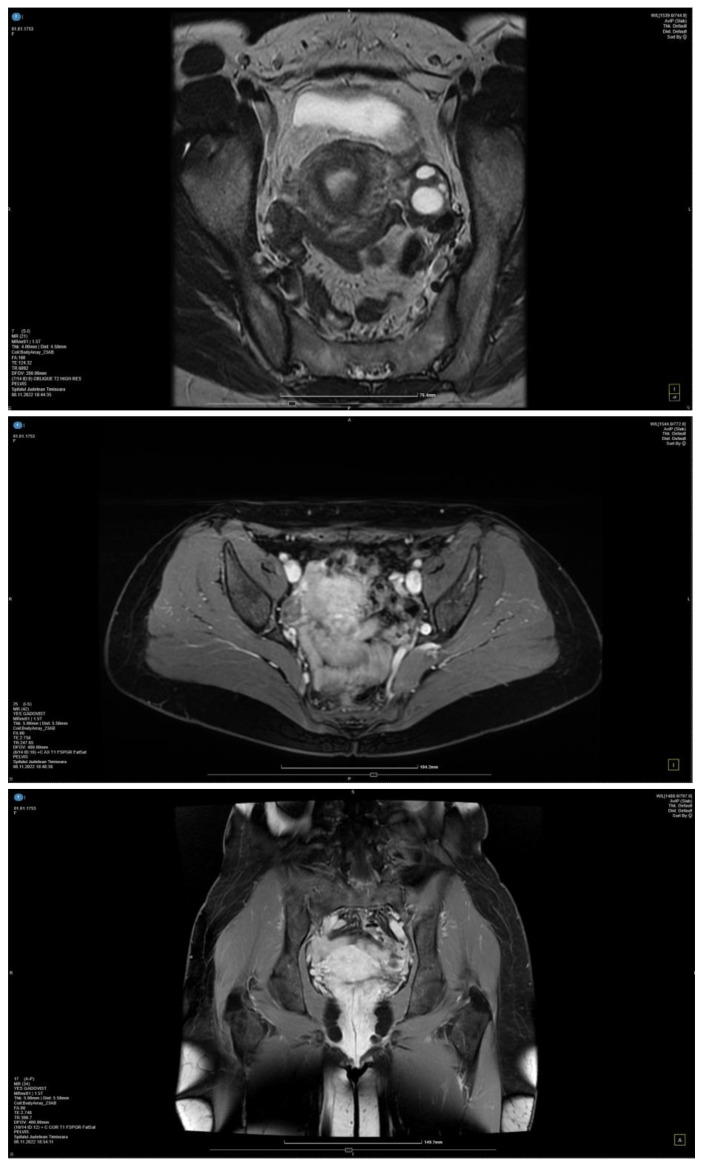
MRI with contrast material in 2 different planes: axial (I) and coronal (A) planes.
